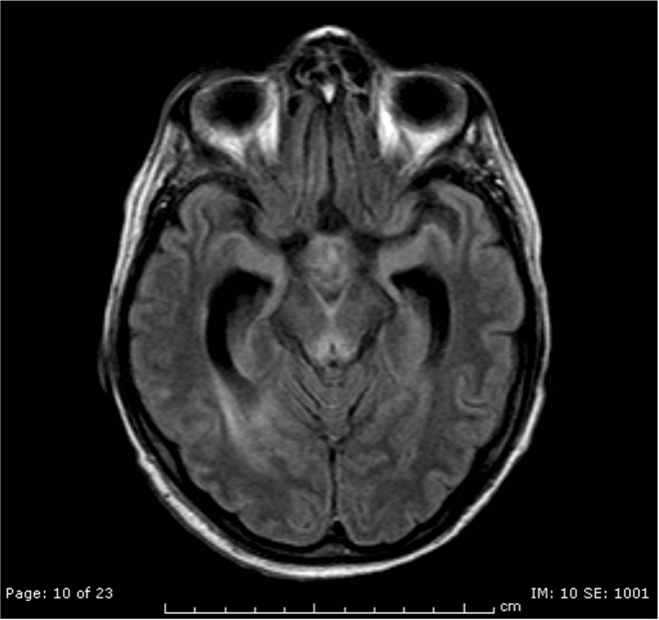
FLAIR axial shows hyperintensity of the periaqueductal grey.
